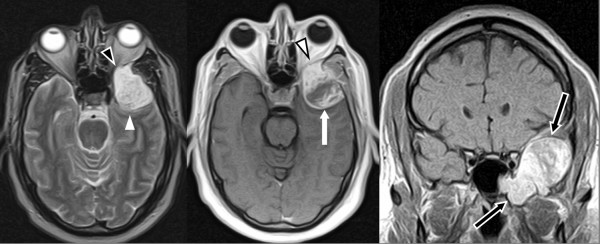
Axial T2-weighted magnetic resonance image demonstrating a mildly heterogeneously hyperintense mass extending from the left middle cranial fossa (white arrowhead) through the left greater sphenoid wing into the left orbit (outlined black arrowhead). The axial T1-weighted gadolinium-diethylenetriaminepentaacetic acid (Gd-DTPA)-enhanced image demonstrates identical findings (white arrow and outlined white arrowhead, respectively) and heterogeneous enhancement of the mass. The coronal T1-weighted Gd-DTPA-enhanced image demonstrates the mass extending downward and medially into the pterygoid recess of the left sphenoid sinus (outlined black arrows).
